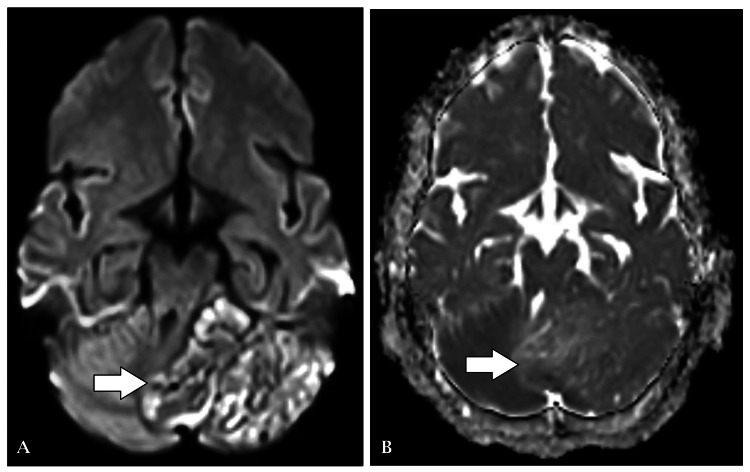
(A) DWI: few areas show restriction. (B) ADC shows a corresponding low signal DWI: diffusion-weighted imaging; ADC: apparent diffusion coefficient
